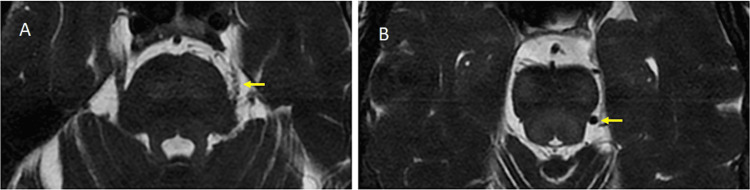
MRI T2 SPACE sequence of the cerebellopontine angles (A) The image shows vascularity along the surface of the left trigeminal nerve; (B) The image shows a dilated left lateral mesencephalic draining vein MRI: Magnetic resonance imaging; SPACE: Sampling perfection with application-optimized contrasts using different flip angle evolution
